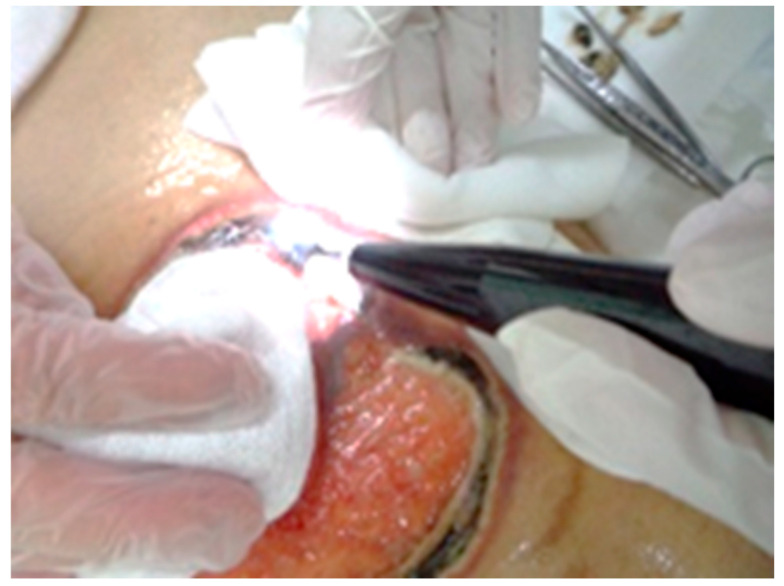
Selective ultrasonic debridement (Week 3–4).
